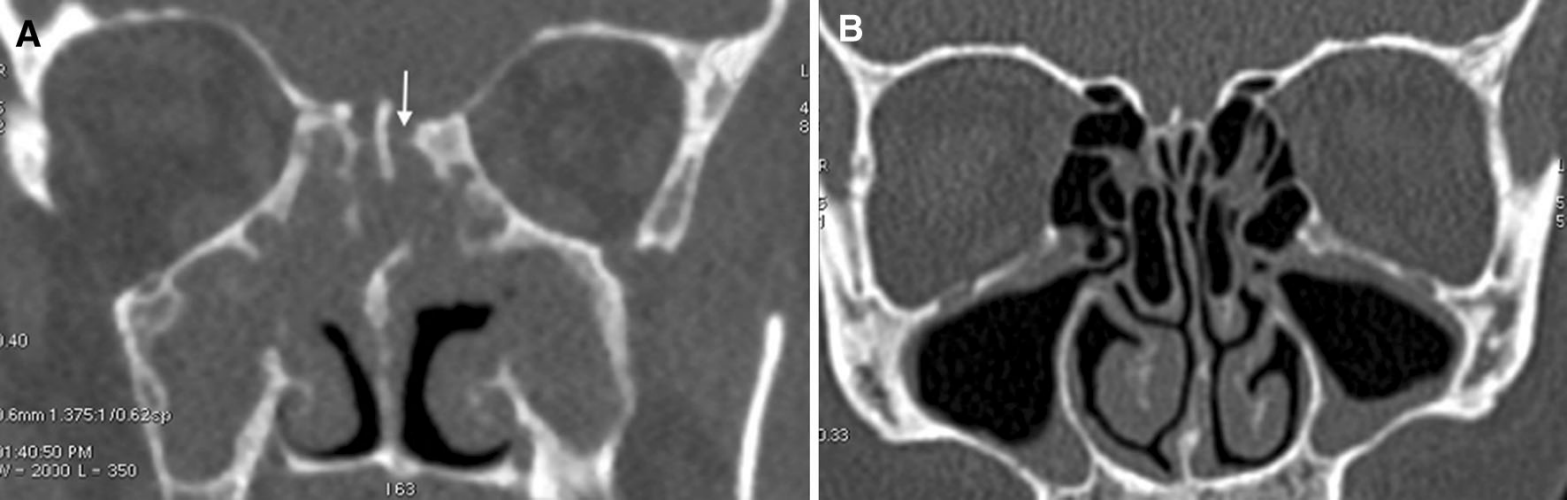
Coronal images of multidetector CT scans obtained according to low-dose (120 kVp, 45 mAs) protocol in patients with chronic rhinosinusitis. Significant image quality deterioration (a) seen i.a. in olfactory fossa (*arrow*) in anosmic subject with massive nasal polyposis (>2 acc. Lildholdt’s scale). Well-defined bony margins (b) in normosmic patient without polyps in nasal endoscopy
